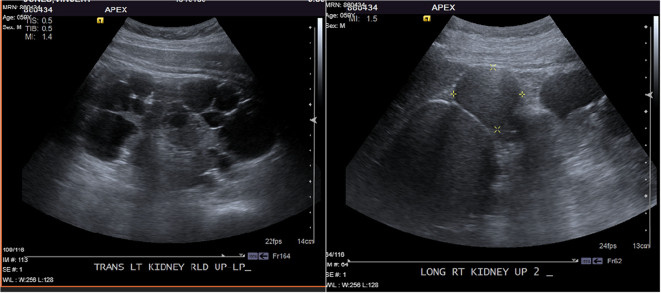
Abdominal ultrasound images of renal cysts in patient. Multiple cysts were noted in the left kidney and some of which were complex. The largest and most complex one measured 7.3 cm. The largest cysts in the right kidney were located in the lower pole with a size of 4.2 cm.
